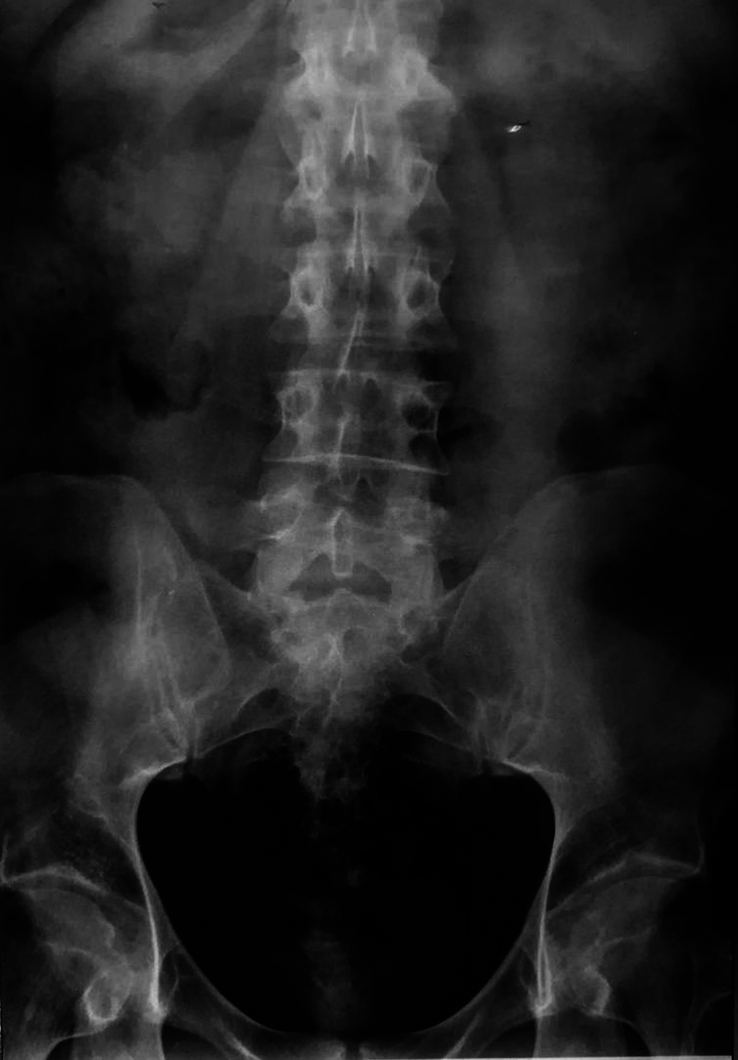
Pelvic X-ray showed bilateral grade IV sacroiliitis.
